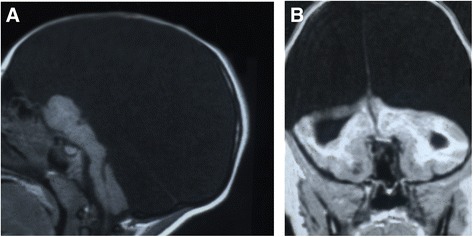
AB: Sagittal (A) and choronal (B) projections of cerebral T1 weighted MRI performed on a patient affected by hydranencephaly. The cerebral hemispheres are completely missing, replaced by a membranous sac filled with cerebrospinal fluid. Skull, brainstem and basal ganglia are preserved.
